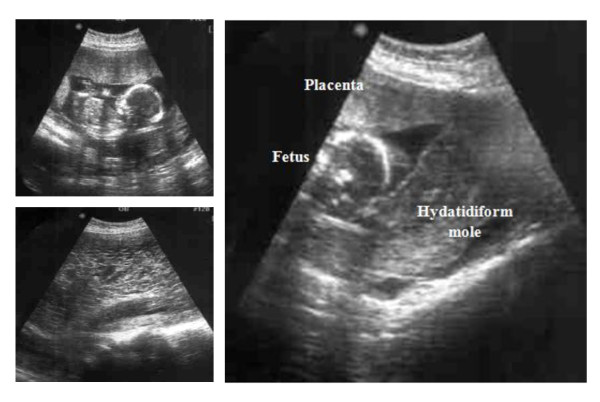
Ultrasound picture of complete hydatidiform mole with coexisting fetus and placenta.
